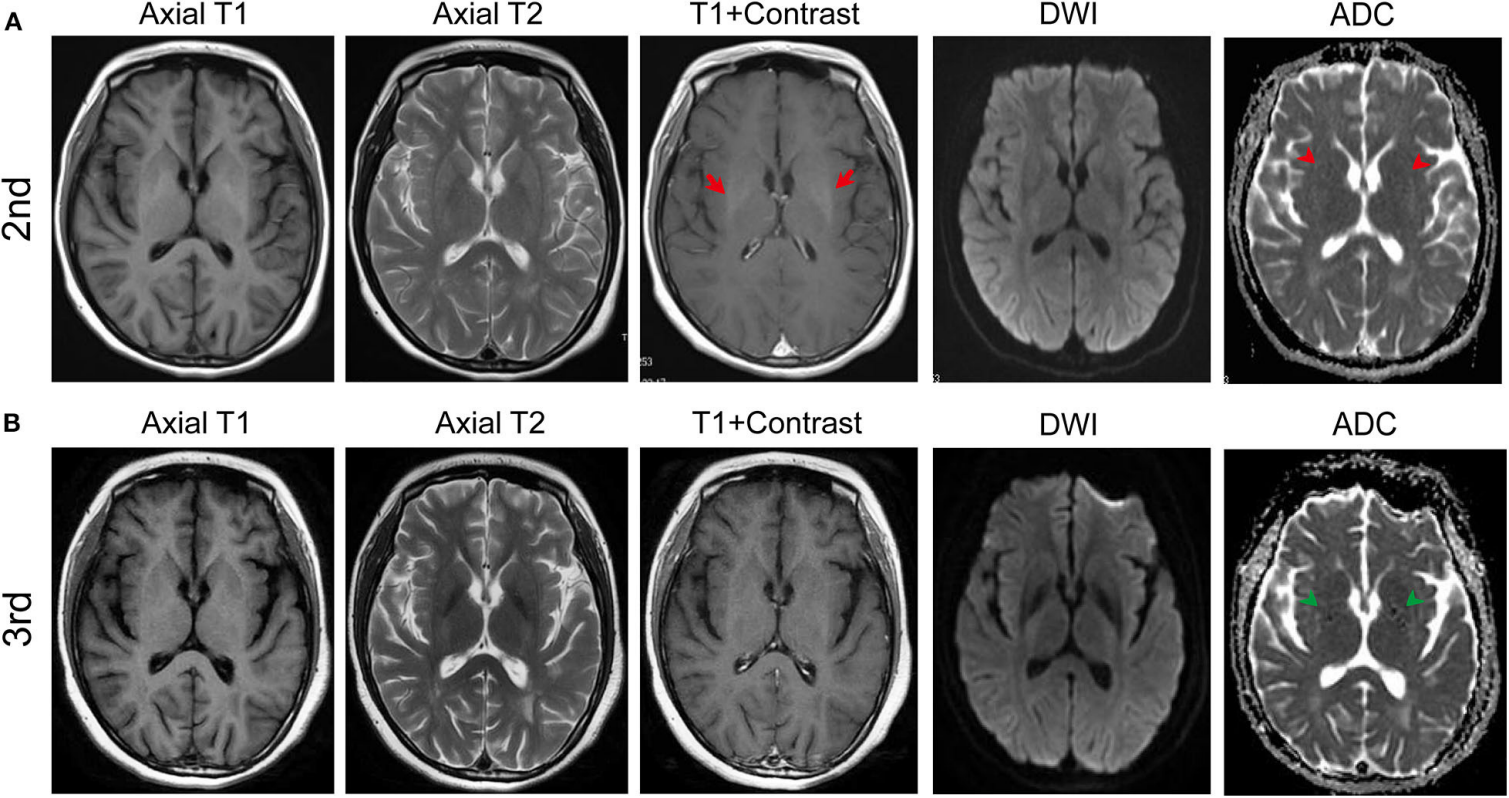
MRI results of the second (in January) and the third admission (in Jun). (A) T1, T2, enhanced T1, DWI, and apparent diffusion coefficient (ADC) axial images in January (2nd). After the immune therapy, the follow-up T1 axial image in January revealed symmetric iso-intensity but enhanced slightly (red arrows) in the bilateral basal ganglion. Dotted hypo-intensity of ADC in the internal capsule (red arrowheads) was noticed. (B) Follow-up MRI in June (3rd) showed the iso-intensity of the bilateral basal ganglion with no enhancement and the dotted hypo-intensity lesion in the internal capsule (green arrowheads).
